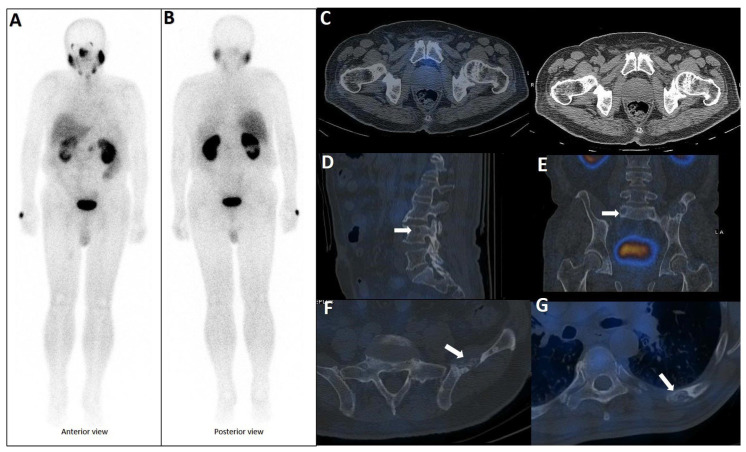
PSMA scan ruling out the possibility of metastatic prostate cancer. A 73-year-old man was referred for diagnosis/staging, due to mildly elevated serum PSA levels, and a few lytic–sclerotic bone lesions incidentally found in the recent abdominopelvic CT scan. Whole-body 99mTc-PSMA (A,B) and SPECT/CT (C) showed faint PSMA uptake in the prostate gland (PRIMARY score 1) with multiple non-PSMA-avid (white arrows) lytic–sclerotic changes in the L4 vertebra (D), sacrum (E), left iliac (F) and left 6th rib (G). No nodal or visceral involvement was evident. These bone lesions were finally concluded as unrelated pathology to prostate cancer, yet the rare possibility of non-PSMA-avid tumor could not be entirely ruled out.
